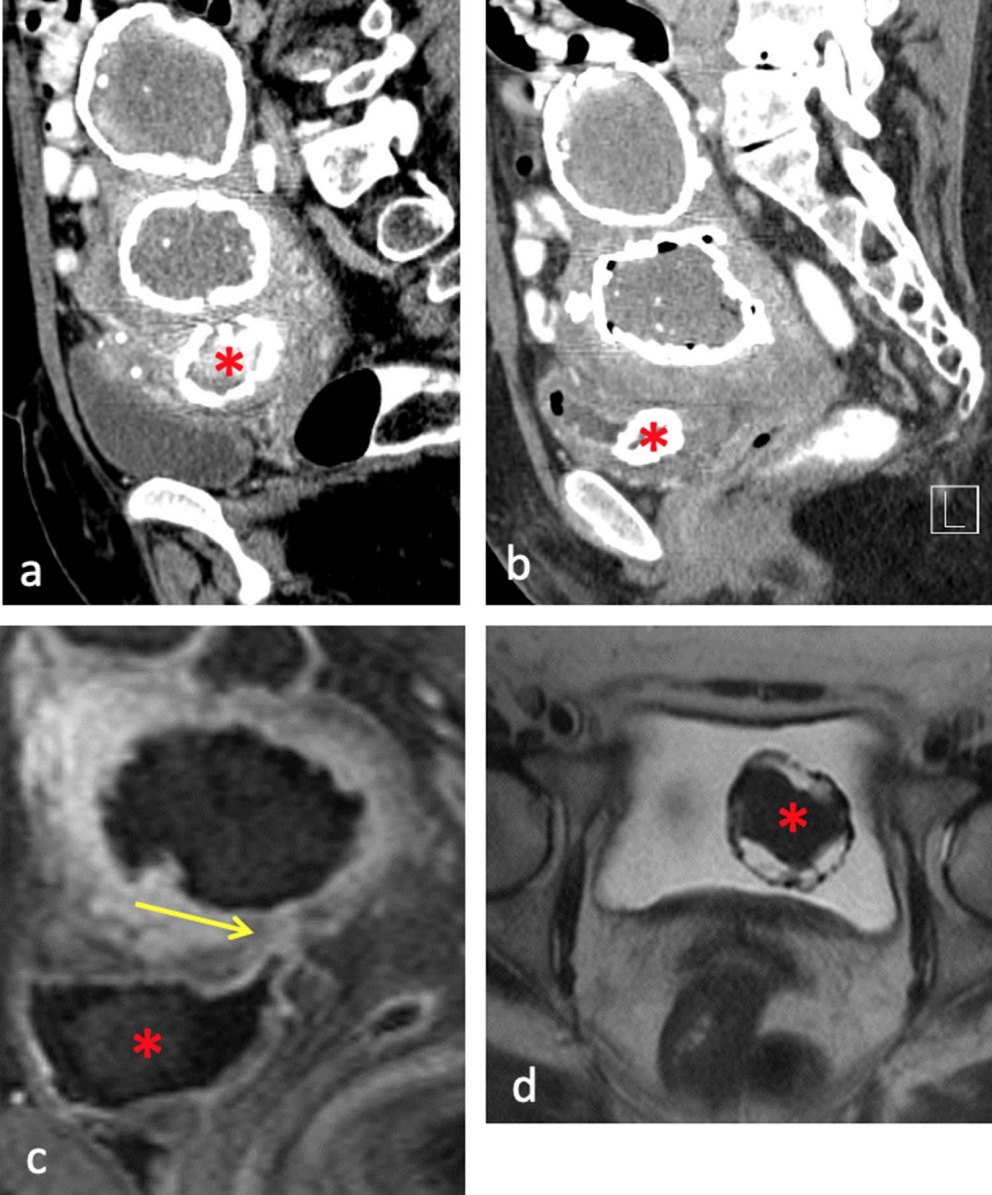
64-year-old female with known calcified uterine fibroids a developed dysuria. Repeat CE-CT b shows interval erosion of the fibroid (*) into the bladder with wall thickening, mucosal enhancement and foci of air. Post-contrast T1-weighted c and T2-weighted (d) images confirm fibroid erosion into the bladder and fistulous connection between the uterus and bladder dome (arrow), compatible with vesicouterine fistula. Patient underwent fibroid removal, bladder reconstruction and hysterectomy
